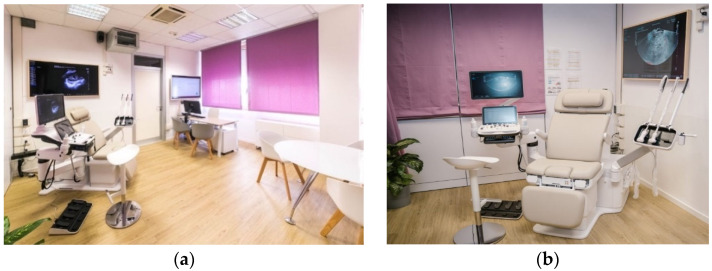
(a,b) Interior view of CLASS Ultrasound.
